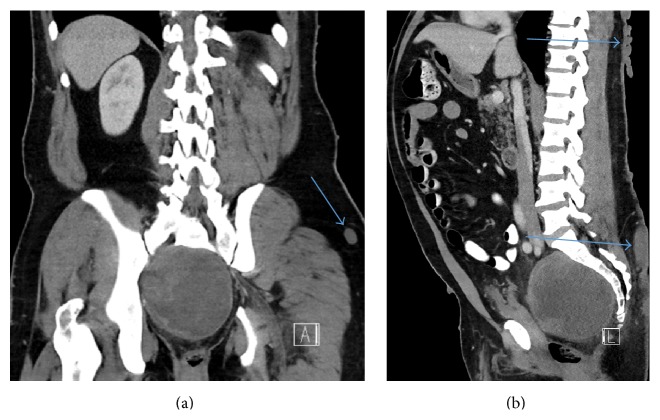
(a) Coronal CT shows heterogeneous pelvic mass and a left hip subcutaneous neurofibroma (arrow) which has the same attenuation as muscle. (b) Sagittal CT shows heterogeneous pelvic mass and numerous posterior subcutaneous neurofibromas (arrows) which have the same attenuation as muscle.
